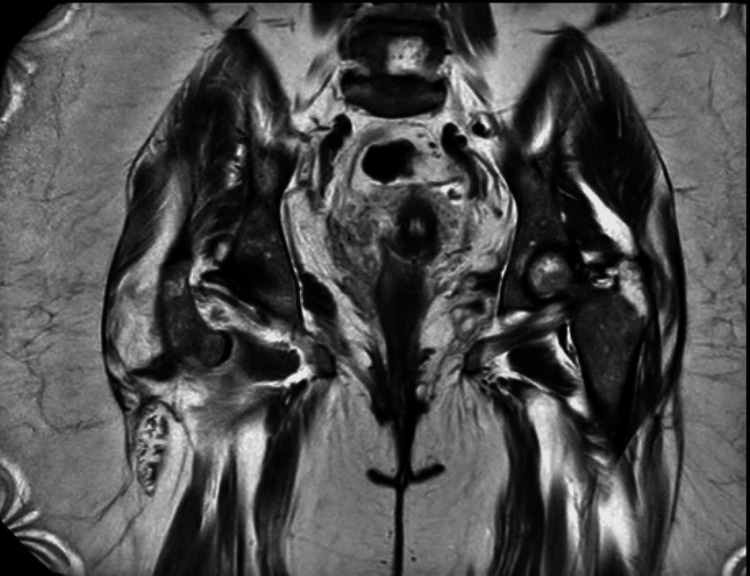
MRI (coronal view) of the pelvis showing extensive soft tissue oedema and swelling centred at pubic symphysis and extending along bilateral adductor muscles, lateral to greater trochanter and along the iliacus muscle within the pelvis (emphysematous cellulitis/fasciitis) There was no drainable abscess.
